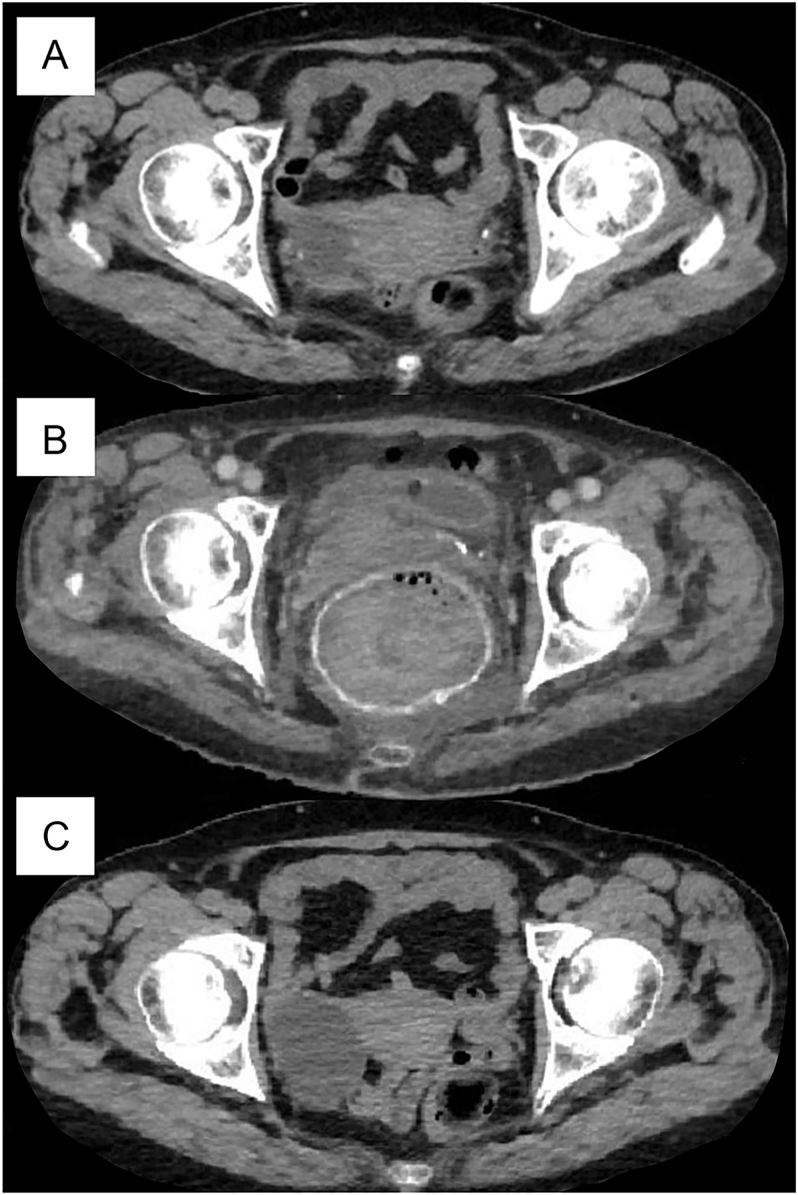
Abdominal CT imaging: almost 3 weeks before the surgery (A), the next day after parathyroidectomy (B), and 2 weeks after parathyroidectomy (C).
